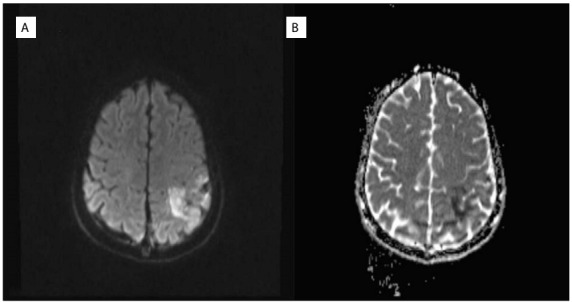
a) Diffusion magnetic resonance imaging; b) Apparent diffusion coefficient.
